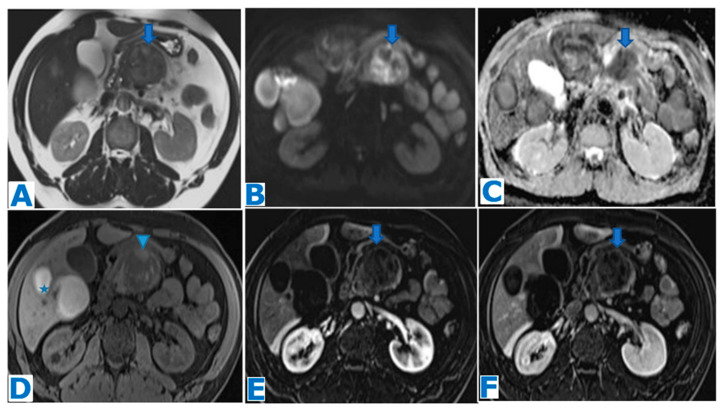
(A): axial T2WI, (B): DWI, (C): ADC, (D): NCE FST1W1, (E): CE arterial phase FST1W1 subtraction, (F): CE portal venous phase FST1W1 subtraction. PM from a known malignant skin melanoma in a 54-year-old patient who presented with acute abdominal pain. A heterogeneous mass was found within the body of the pancreas (arrow in (A)), showing diffusion restriction (arrow in (B,C)) and no pancreatic duct dilatation. The lesion showed hyperintense content in T1WIs (arrowhead in (D)) compatible with melanin, with scarce enhancement after intravenous contrast administration (arrow in (E,F)). There were also several melanin-containing hepatic lesions (* in (D)). Diagnosis of pancreatic and hepatic metastases was proven by biopsy.
